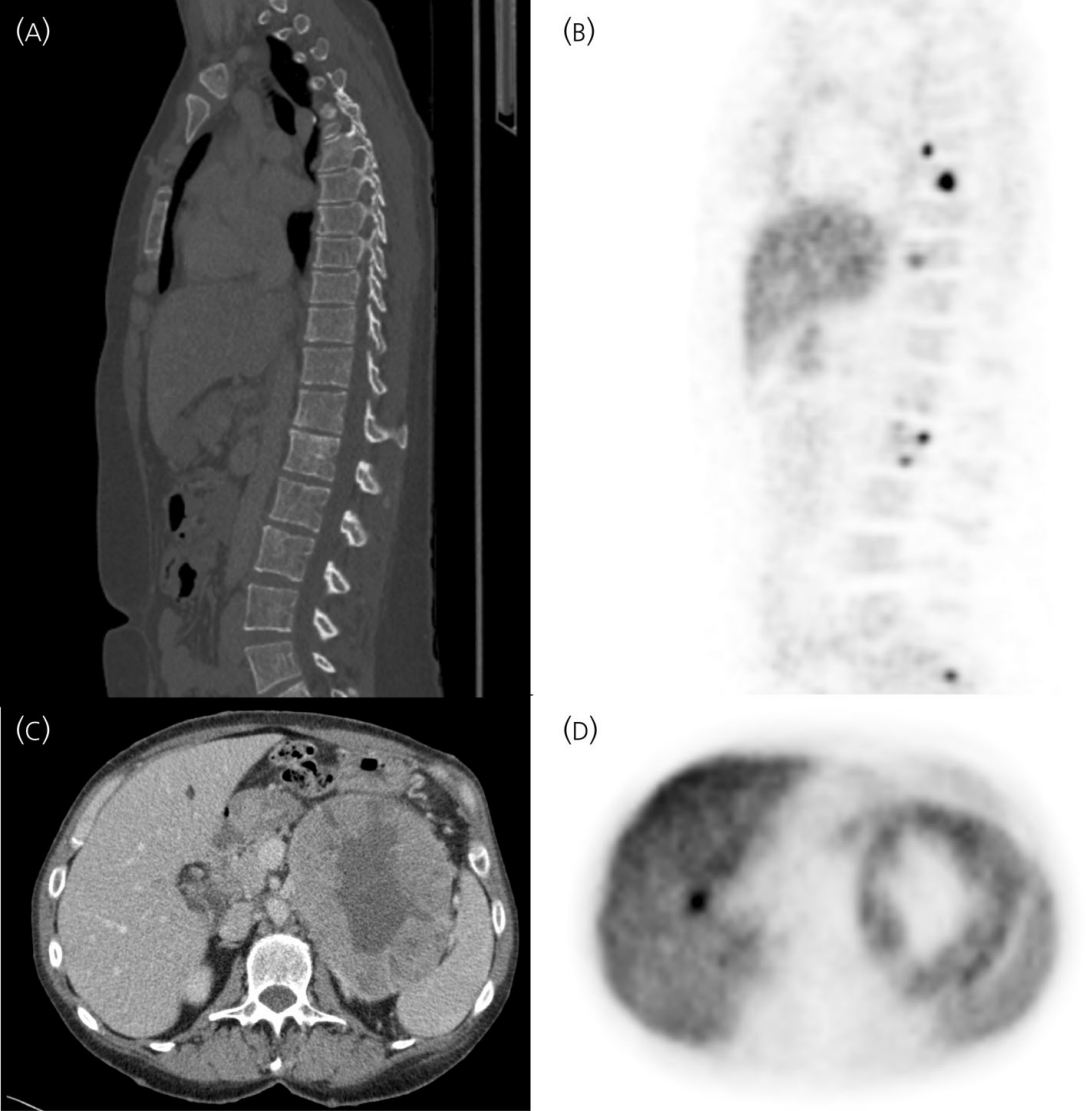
CT and HED‐PET/CT imaging results. (A) CT, in Patient 14, with no evidence of bone metastasis. (B) HED‐PET/CT, in Patient 14 showing multiple metastatic lesions. (C) In Patient 18, the preoperative CT scan shows a large primary pheochromocytoma but fails to identify the liver metastasis. (D) HED‐PET/CT scan show a liver metastasis with distinct tracer uptake.
